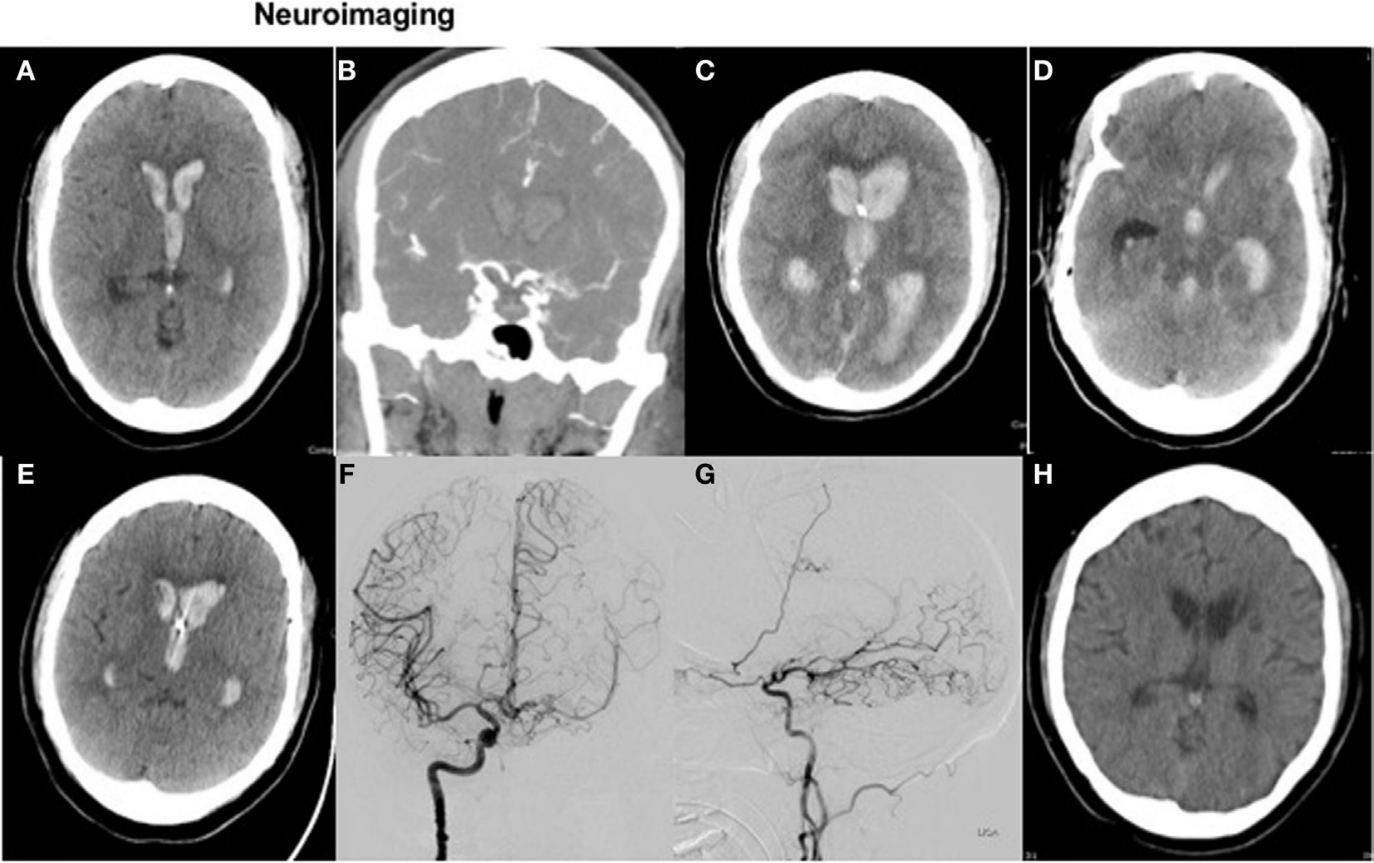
Neuroimaging. Figure 1 with axial CT head (A) and coronal CTA (B) on presentation, with repeat axial CT 3 h later (C,D) and axial images on posthemorrhage day 4 and 21 (E,H). Diagnostic cerebral angiogram (coronal view and sagittal view) is shown in images (F,G), respectively.
